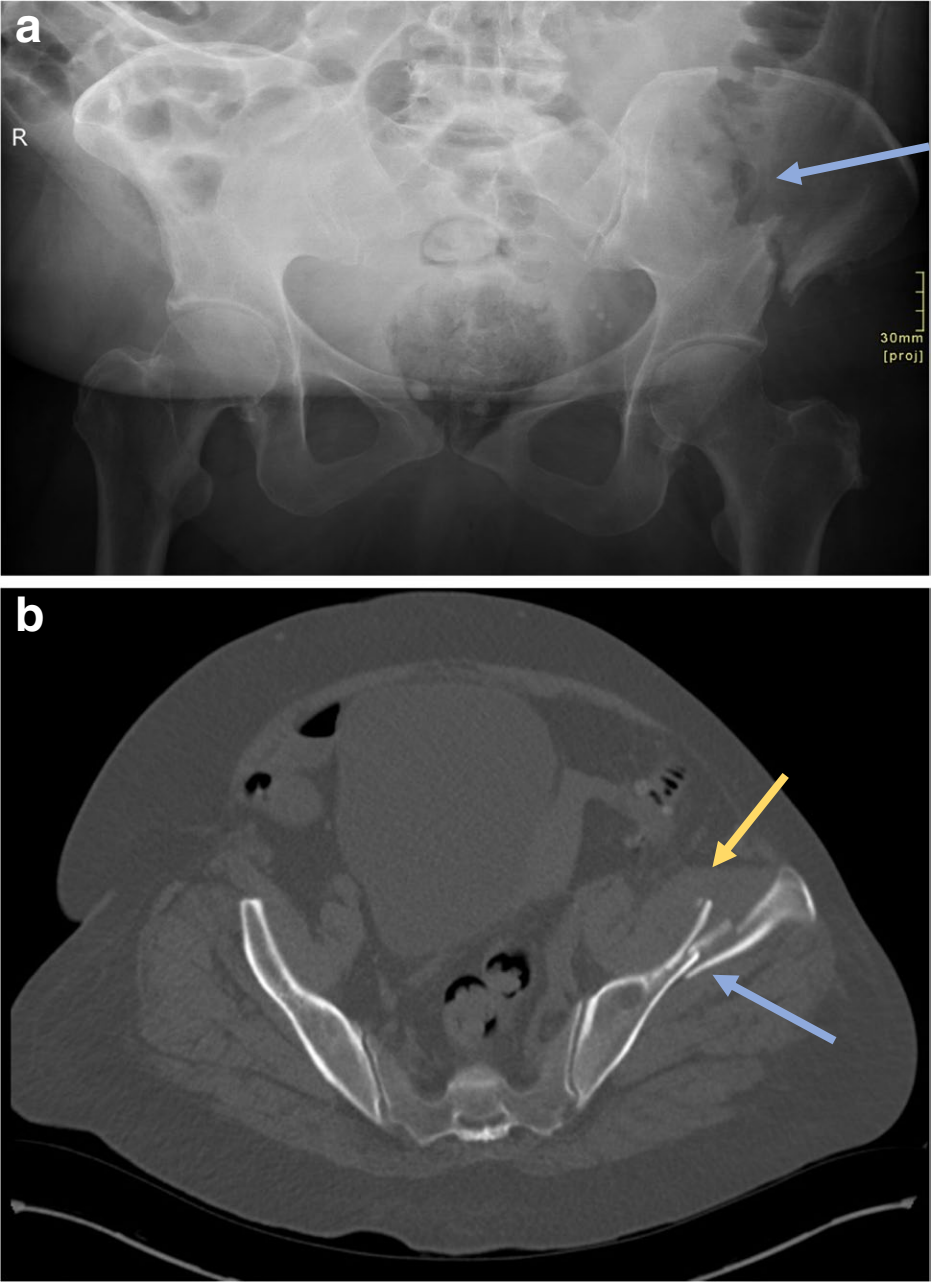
A left iliac wing fracture associated with bisphosphonate use in a 63-year-old woman presenting with lumbar pain radiating to her hip. a Anteroposterior pelvic radiograph showing a vertically orientated, displaced fracture of the left iliac wing (blue arrow). b Axial computed tomography of the pelvis without intravenous contrast showing a left iliac wing fracture (blue arrow) and soft tissue swelling in the overlying left iliacus muscle, likely haematoma (yellow arrow), without appreciable cortical thickening
